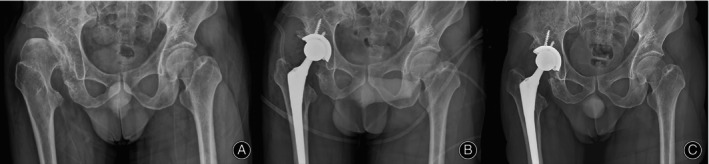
Radiographs of a 55‐year‐old man with right Crowe type III DDH underwent THA with BG technique. (A) Preoperation. (B) Immediate radiograph after THA showed complete coverage of the acetabular prosthesis with bone graft. (C) The radiograph at 3 years postoperatively showed similar bone density between the bone graft and the acetabulum bone, proper prosthesis position, and no prosthesis of loosening or sinking.
